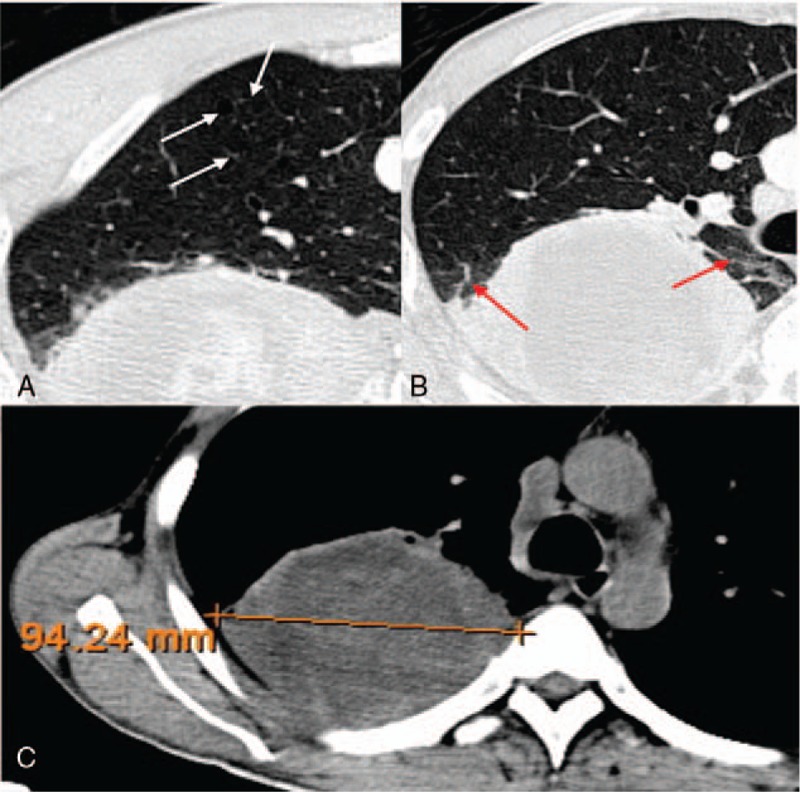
A 55-year-old man with a smoking history of 35 years had right upper lobe lung adenocarcinoma in EGFR wild type, in whom axial CT images show a solid mass with some emphysema (A, white arrow), a litte fibrosis (B, red arrow) and no pleural retraction or Spicule sign, whose maximum diameters were about 94 mm (C).
